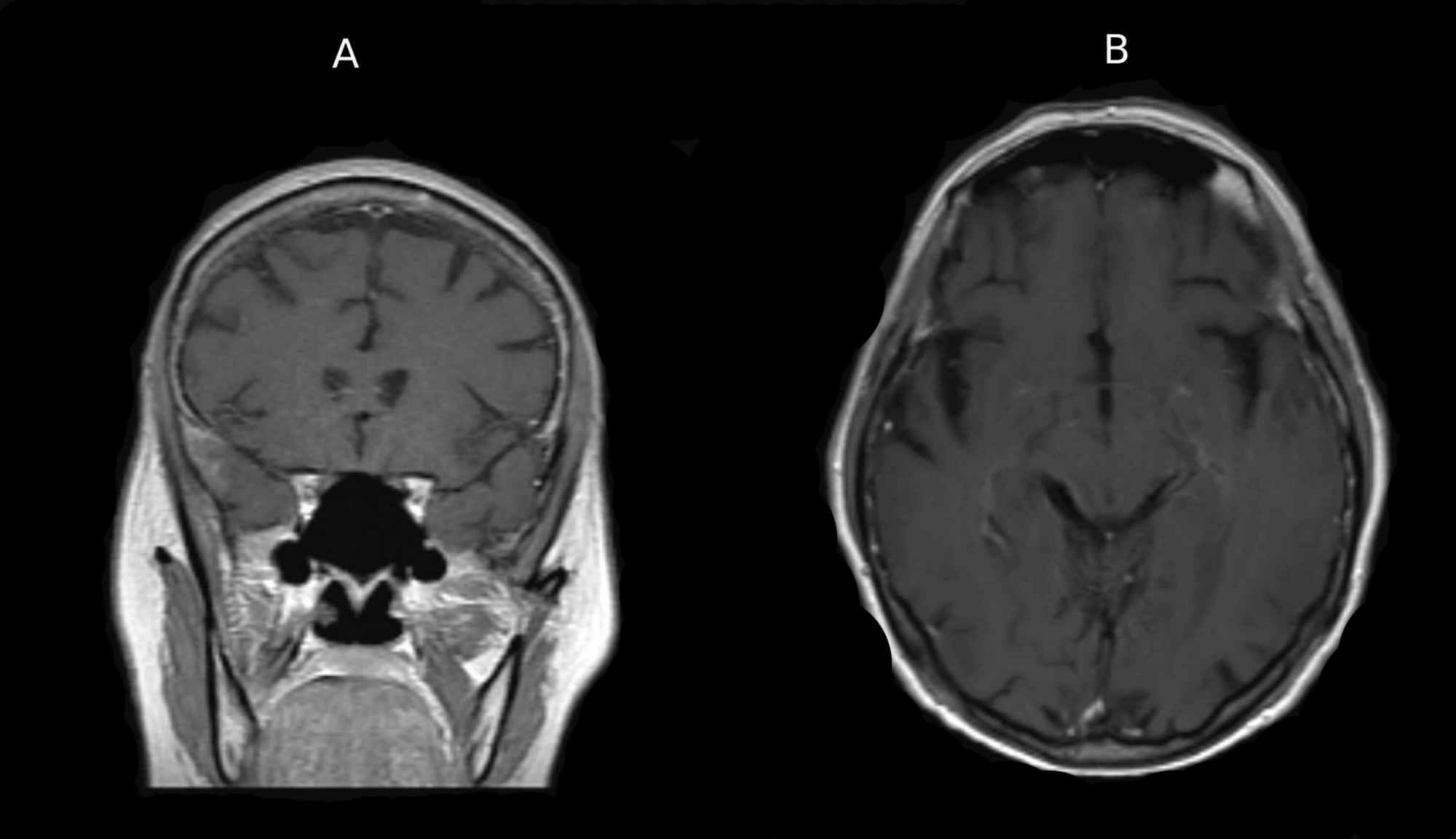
MRI. (A) Coronal view. (B) Axial view. T1-weighted MRI with contrast showing no post-contrast enhancement in the left temporal region in both views. MRI, magnetic resonance imaging
